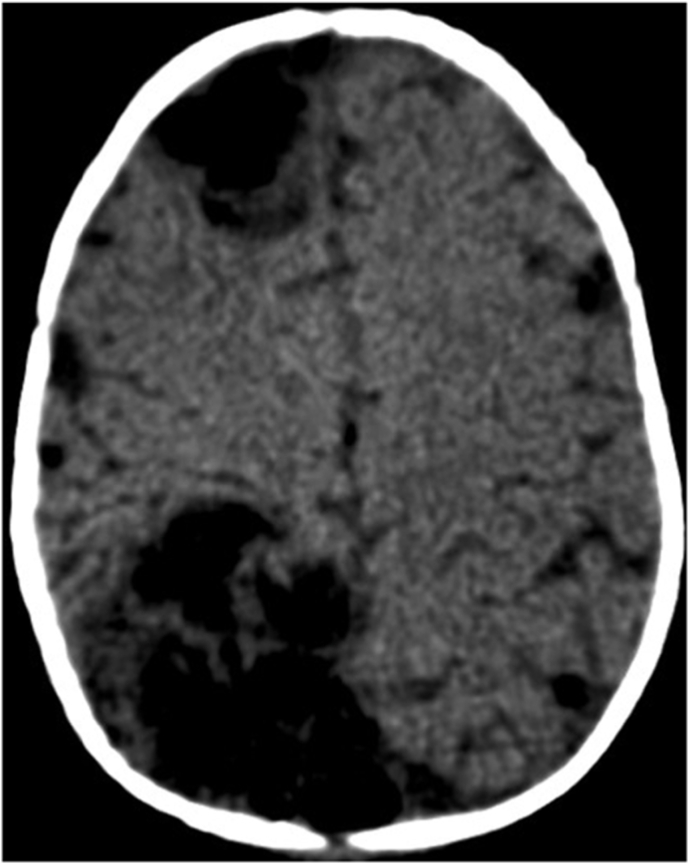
Head CT scan showing a right temporo-parietal hypodense area with suspicion of a cerebral venous thrombosis with no signs of intracranial hypertension.
